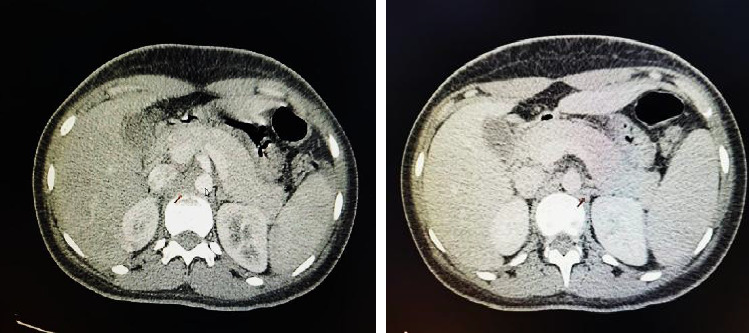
Abdominal CT images in arterial and venous phase showing edematous pancreas and inflammatory changes of the peripancreatic fat.
